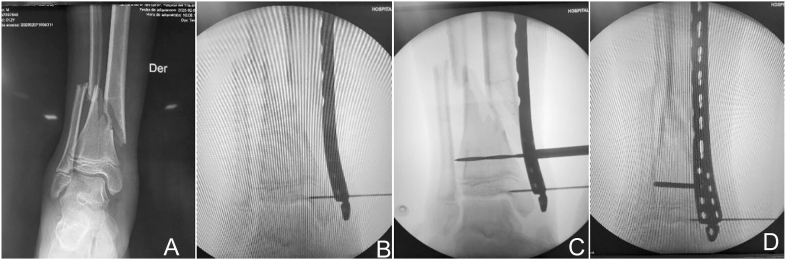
Preoperative anteroposterior radiograph (A), insertion of a temporary pin to hold the plate in place (B), bone drilling with a drill bit (C), and fracture reduction using a screw to approximate the bone fragment to the plate (D).
